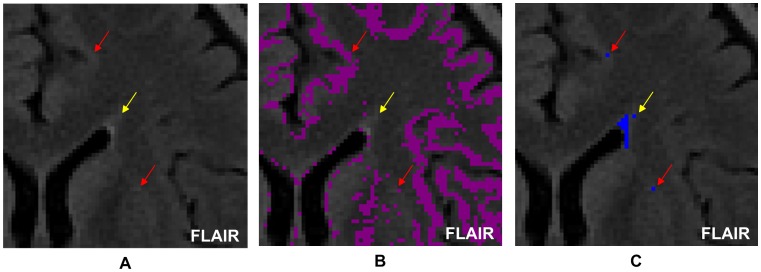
Removal of hyperintensive junction-connected regions from candidate WMHs. (A) The three arrows point to three hyperintensities on a FLAIR image; (B) Shown in purple is the gray/white matter junction created in Step 10.1. This figure shows that the two hyperintensities pointed to by the red arrows were junction-connected, whereas that pointed to by the yellow arrow was not junction-connected; (C) The three hyperintensities were all nominated as candidate WMHs in Step 9. However, in Step 10.2, the two pointed to by the red arrows would be eliminated from the candidate WMHs and only the one pointed to by the yellow arrow would remain in the candidate WMHs.
